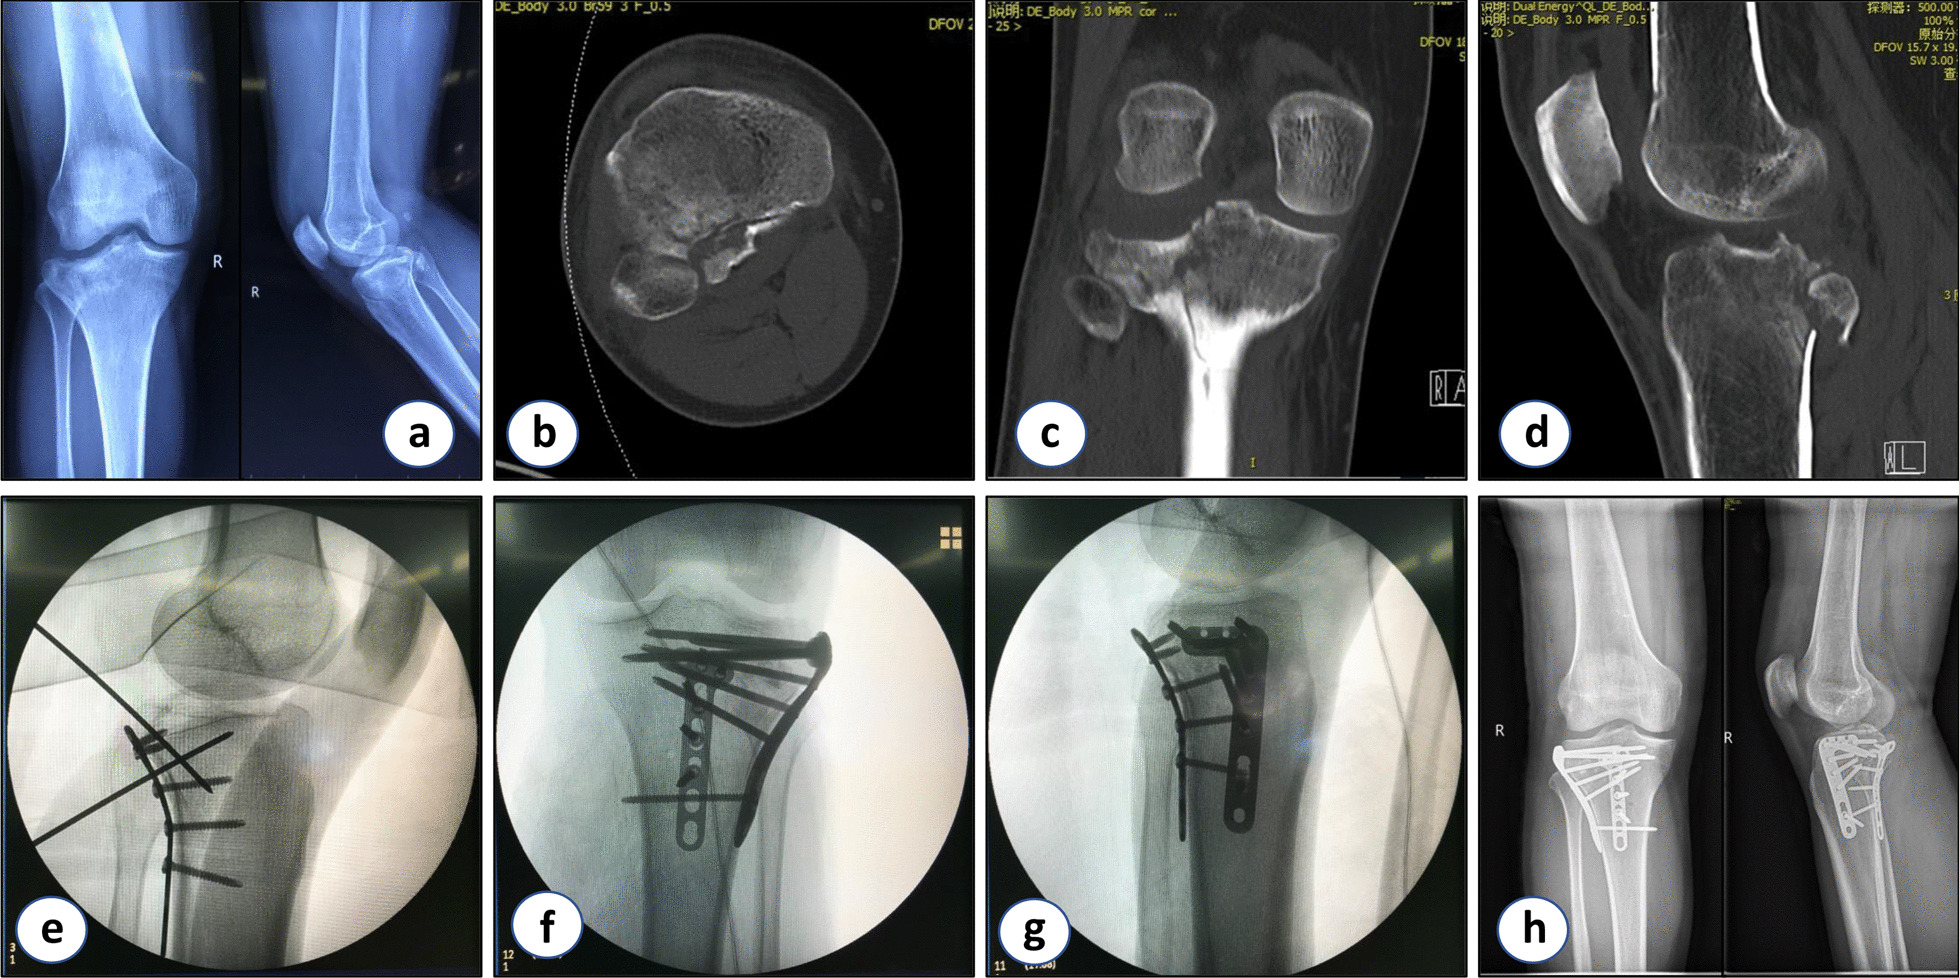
A patient with type II flexion-valgus tibial plateau fracture. a Preoperative X-ray examination of the knee joint. b Preoperative transverse CT image of the tibial plateau. c Preoperative coronal CT image of the tibial plateau. d Preoperative sagittal CT image of the tibial plateau. e–g Intraoperative C-arm fluoroscopy. h Postoperative X-ray examination of the knee joint
